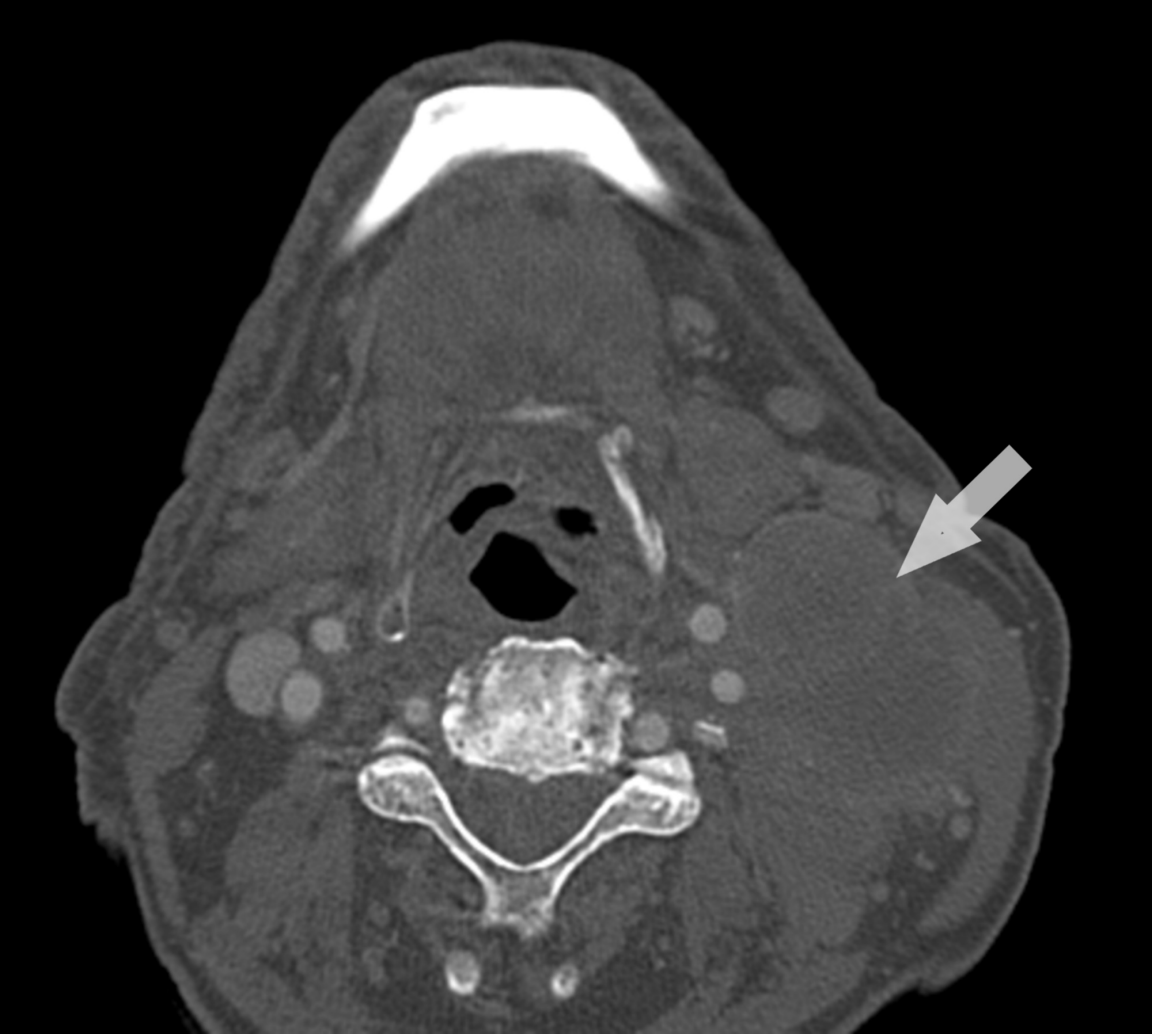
Cervical CT-scan at the time of diagnosis (axial view)
